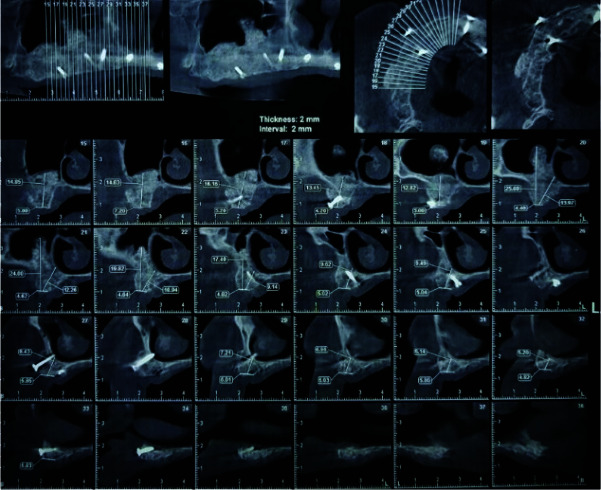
Cone beam computed tomography (CBCT) 6 months postoperatively
